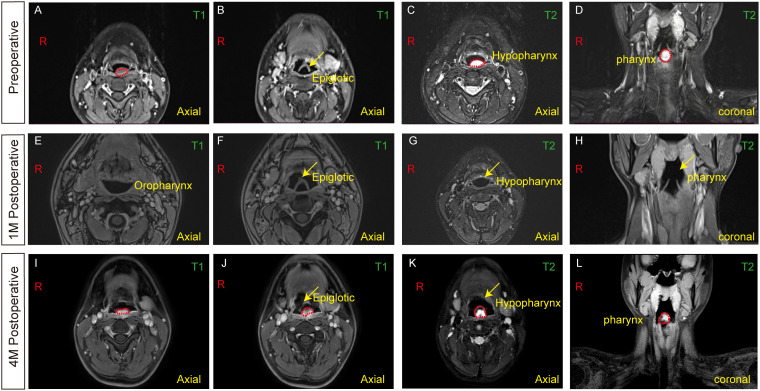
Preoperative and postoperative MRI findings. Preoperative MRI showed a well-circumscribed submucosal lesion in the left hypopharyngeal region. Axial T1-weighted images (A, B), axial T2-weighted image (C), and coronal T2-weighted image (D) demonstrated the lesion adjacent to the epiglottis and hypopharyngeal/pharyngeal region. One month after surgery, axial T1-weighted images (E, F), axial T2-weighted image (G), and coronal T2-weighted image (H) showed postoperative changes without definite residual tumor. Four months after surgery, axial T1-weighted images (I, J), axial T2-weighted image (K), and coronal T2-weighted image (L) demonstrated a newly detected enhancing lesion at the operative site, supporting early local recurrence.
